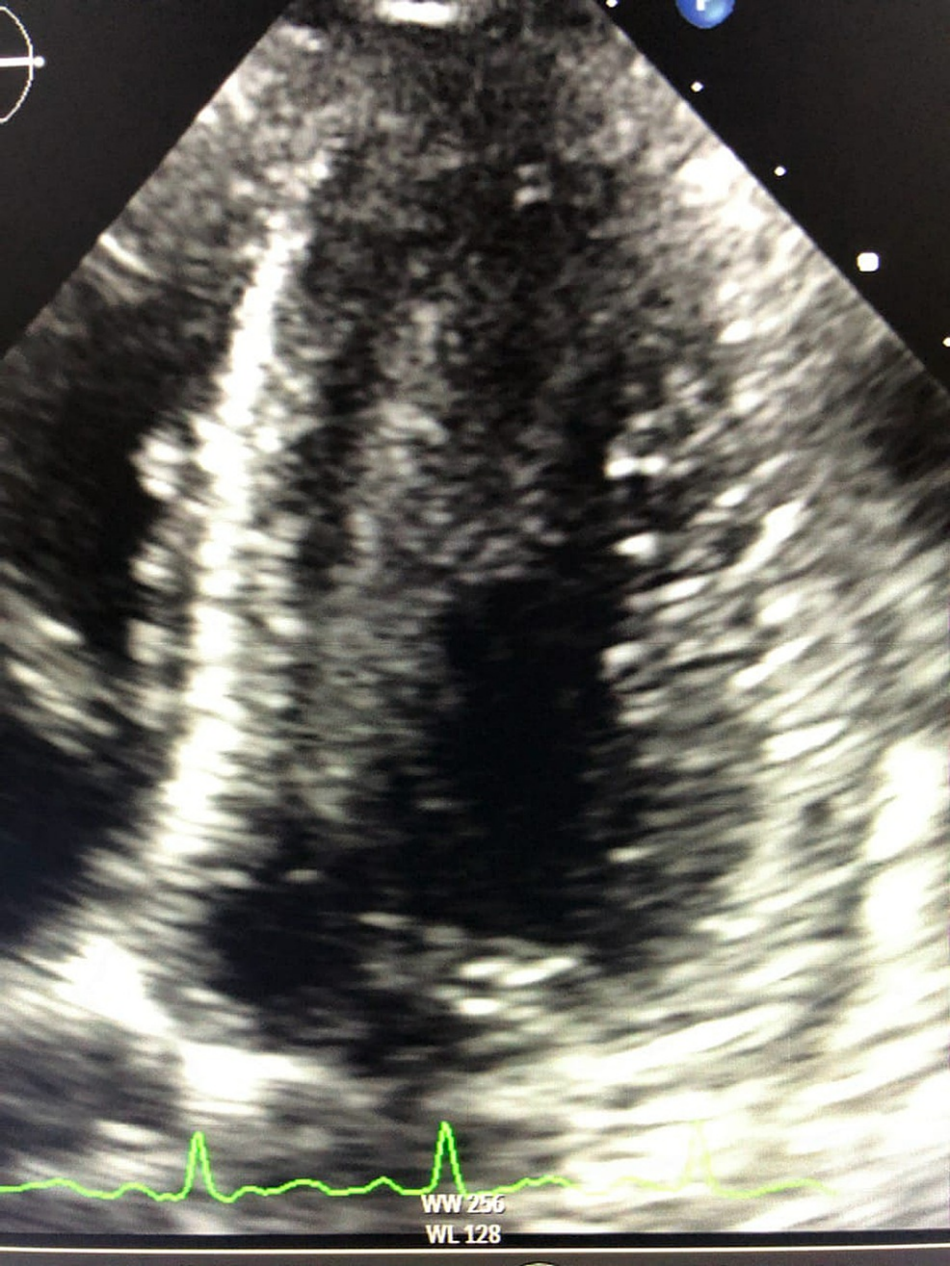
Moderate to severe global hypokinesis of the LV on echocardiogram LV: left ventricle
